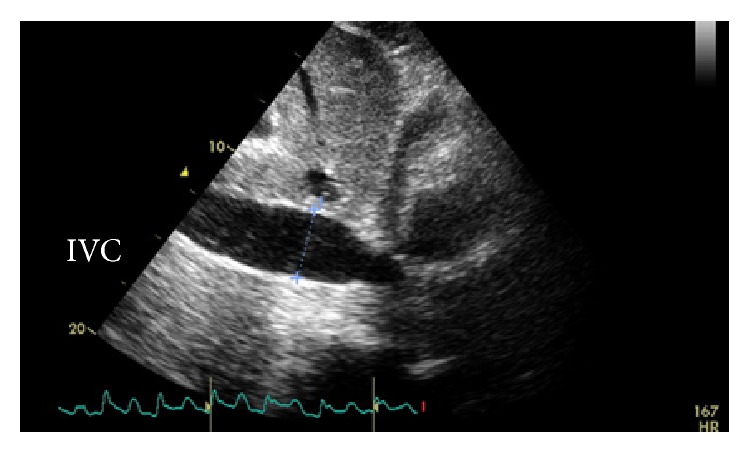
Echocardiographic image showing noncollapsible inferior vena cava with pericardial effusion.
